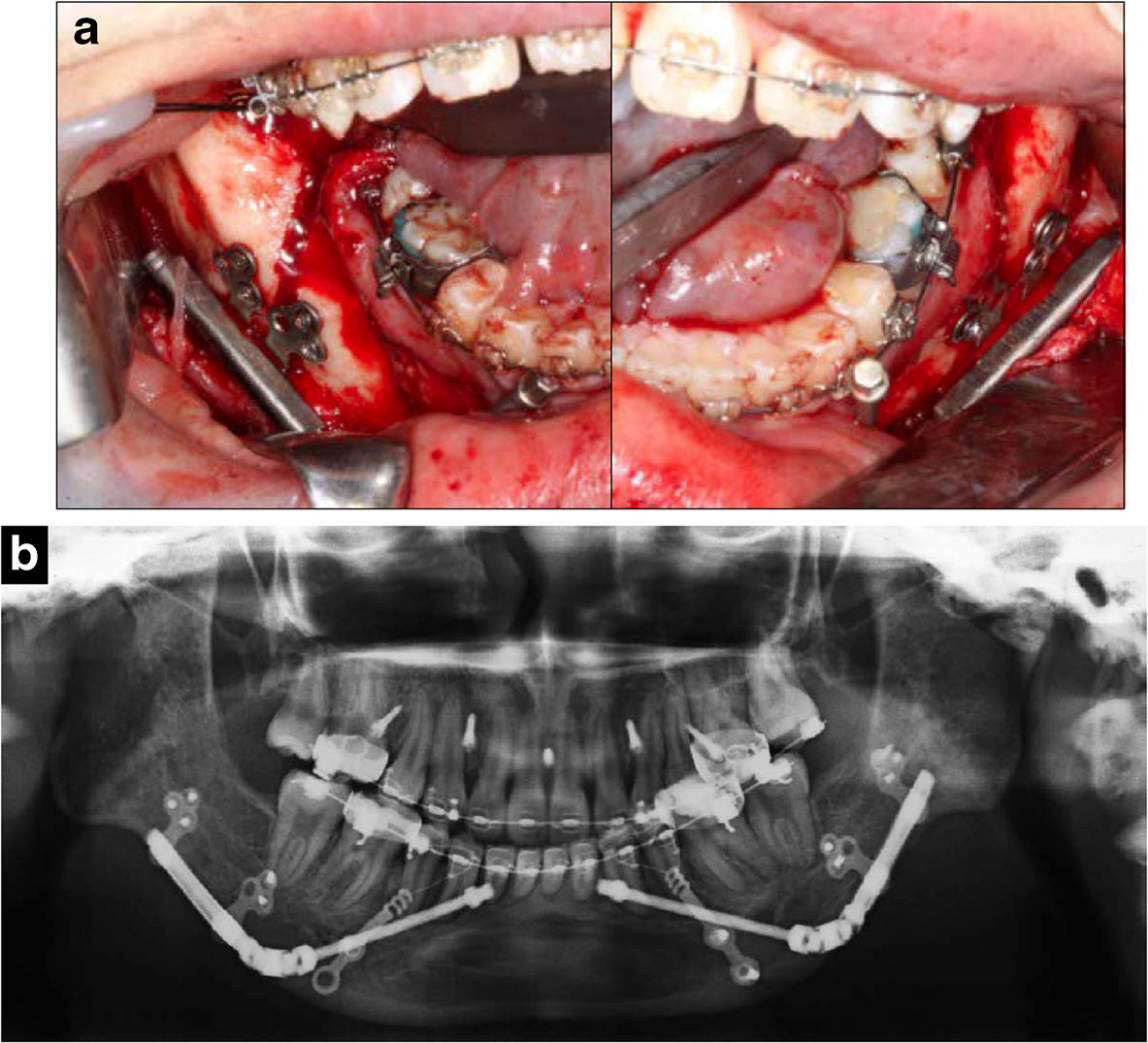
a Distraction devices applied to the posterior region of the mandible body bilaterally. b Panoramic radiograph after mandibular distraction osteogenesis
